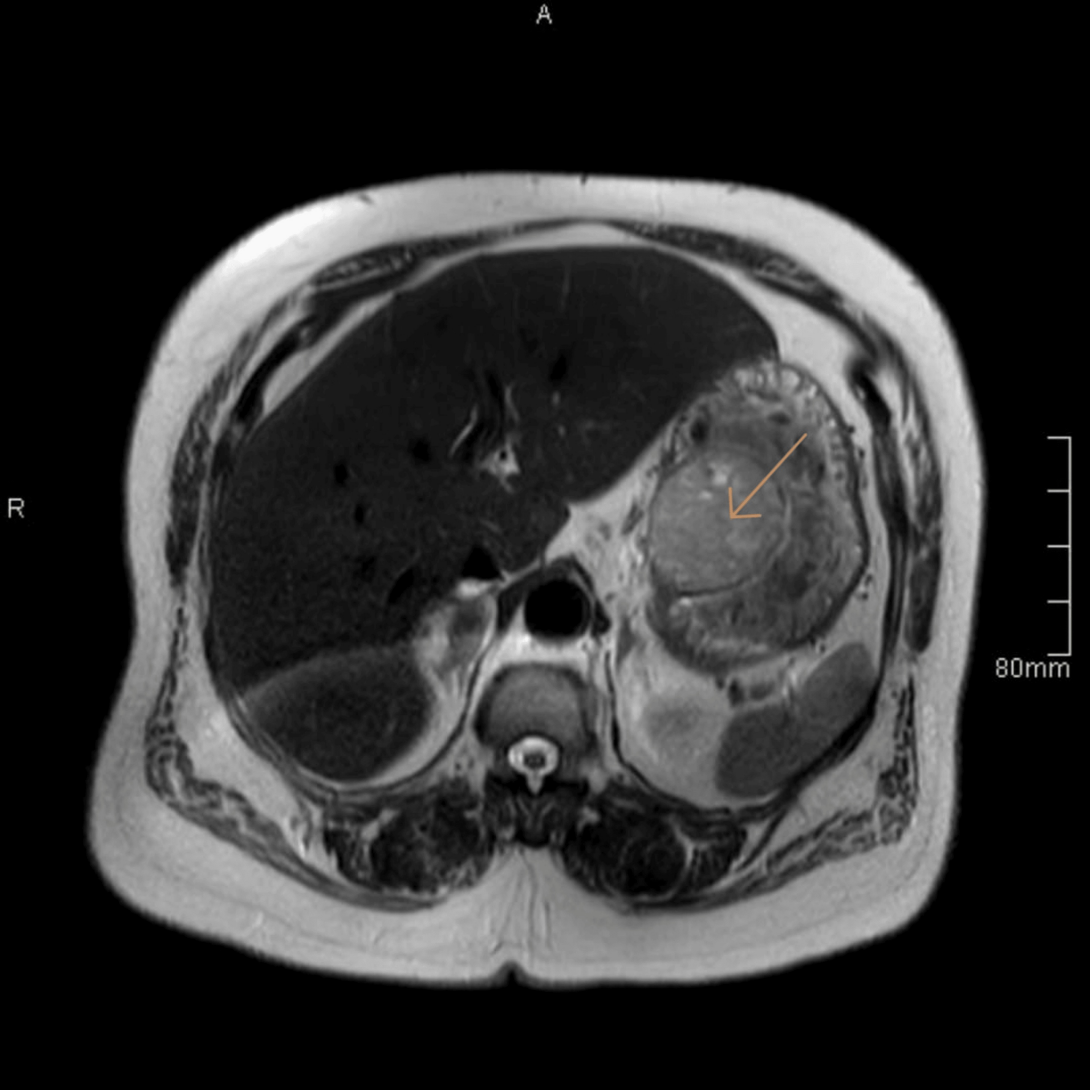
A well-circumscribed, exophytic soft tissue mass lesion arising from the inner surface of posteriomedial gastric wall The lesion shows heterogeneous signal intensity on T2-weighted MRI, with central areas of necrosis or cystic degeneration, and shows no definite invasion or extra gastric extension
